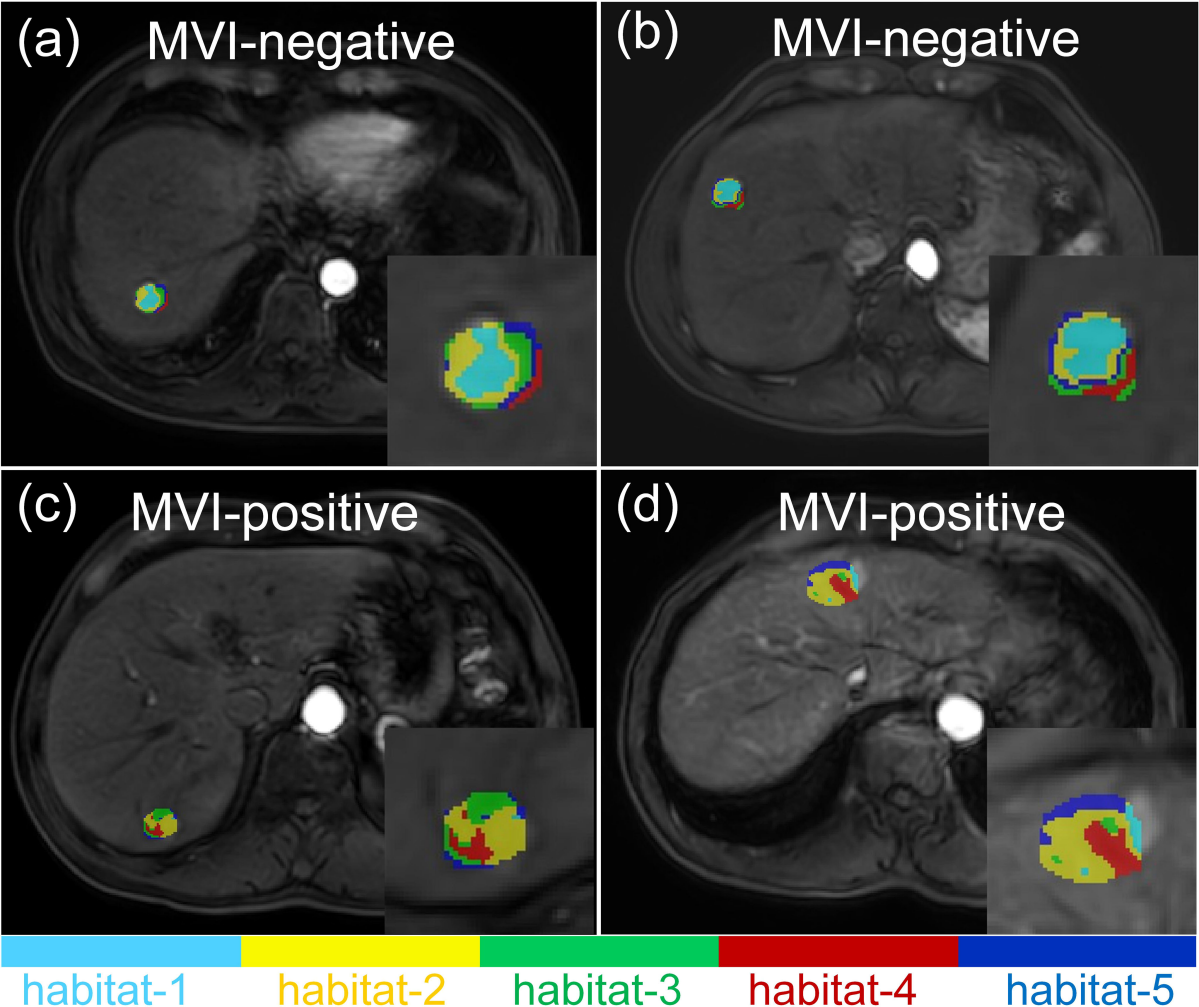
Representative MRI images of two MVI-negative (a, b) as well as two MVI-positive HCCs (c, d).
